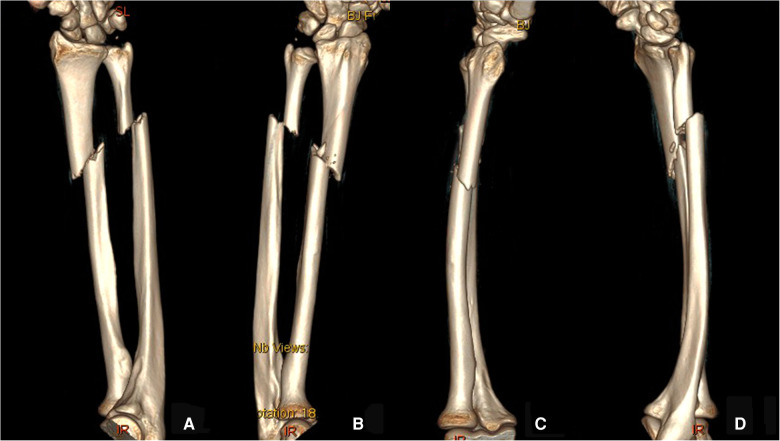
Preoperative three-dimensional reconstructive CT images (A–D) of the left forearm.
